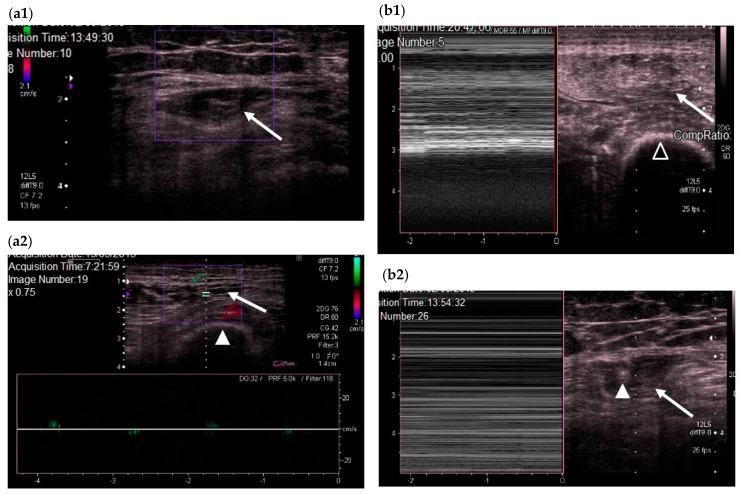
(a) Transversal QRF muscle US scan and color Doppler. (a1) QRF muscle without vascularization (echogenicity Category 3). (a2) QRF muscle with vascularization (echogenicity Category 2): the femur (arrowhead) and the QRF muscle (arrow). (b) Transversal US scans and M-mode; (b1) QRF muscle (arrow) with fasciculations (echogenicity Category 2) and the femur (black arrowhead); (b2) QRF muscle without fasciculations (echogenicity Category 3). QRF tendon of 3.6 mm (white arrowhead) and the QRF muscle (arrow). QRF: quadriceps rectus femoris, US: ultrasound.
